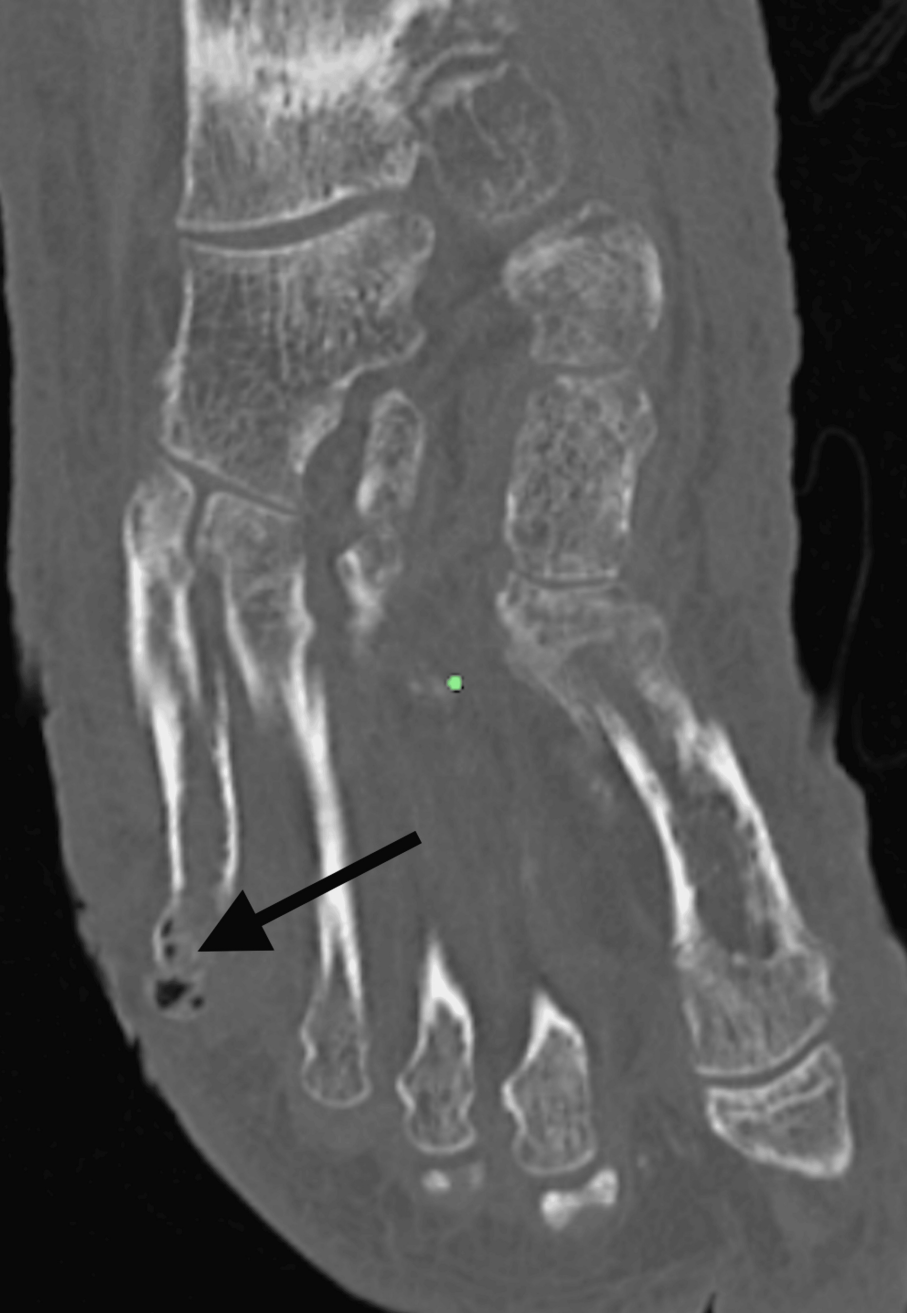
Emphysematous osteomyelitis of the left fifth metatarsal head. A CT sagittal view of the left foot in a 57-year-old man presenting with left lower extremity pain showing emphysematous osteomyelitis.
